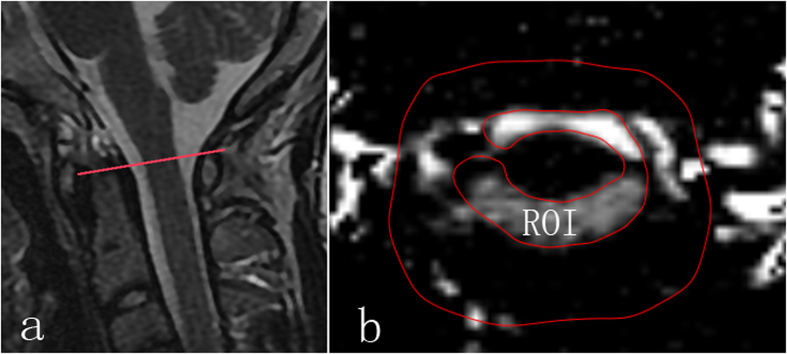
Example of MR images from one of the volunteers. The region of interest was the entire cross-sectional area of the subarachnoid space at the level of the atlas upper border. (a) Showing the level of upper border of the atlas (red line) in the median sagittal plane of head and neck; (b) the region of interesting (ROI, inner closed irregular contour) showing the entire cross-sectional area of the subarachnoid space in the transverse plane at the level of the atlas upper border. And the outer red line served as a guide line.
